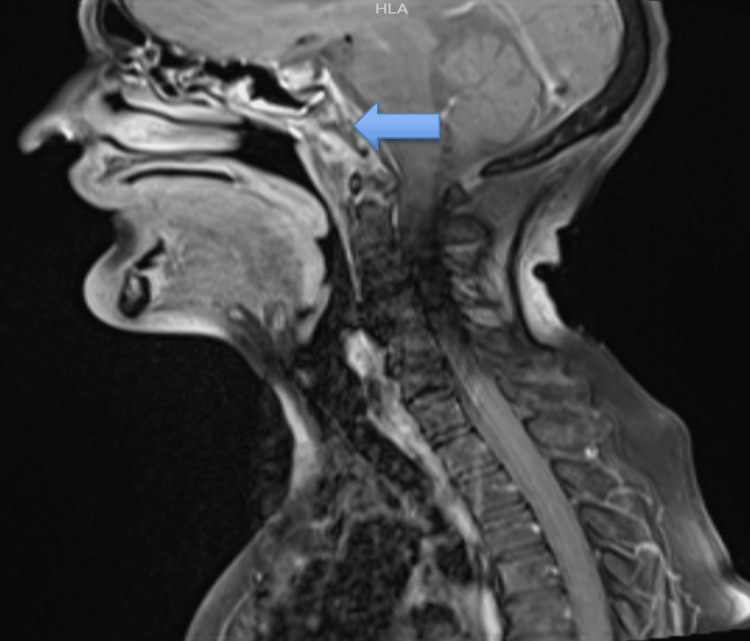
Contrast-enhanced MRI of the brain (T1-weighted) showing post-contrast enhancement of the clivus, and occipital condyles with adjacent peripherally enhancing collection. The blue arrow points to the bone marrow edema in the clivus with diffuse surrounding soft tissue swelling.
